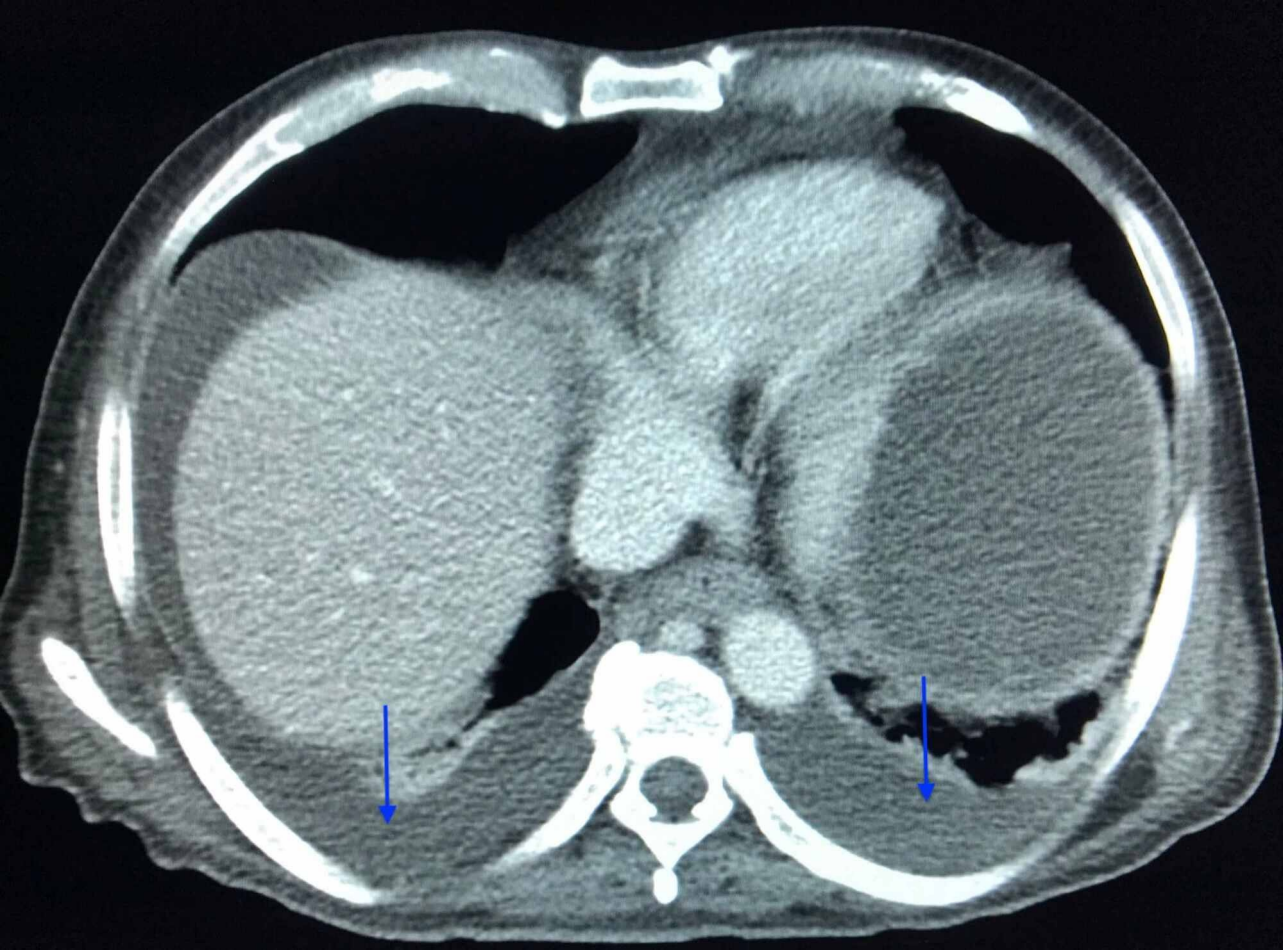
Pleural lung effusions visualized on CT of thorax, transverse plane Pleural effusions are indicated by the blue arrows, as can be seen along the inferior border of the lungs.
